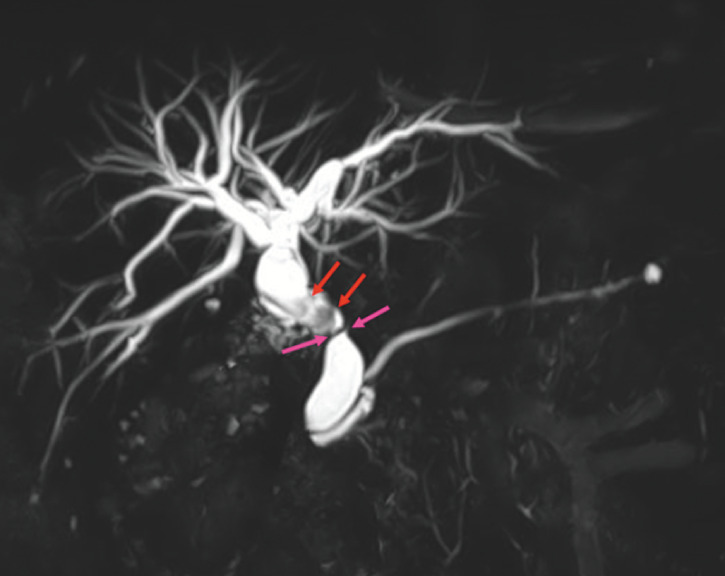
Magnetic resonance imaging (MRI) revealed stones (red arrows) and biliary stricture (pink arrows) in the common bile duct.
